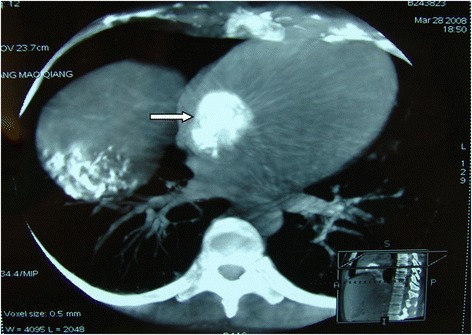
The same patient presented in Fig. 4; C-armed CT showing nice lipiodol deposition in the tumor IVC/RA thrombus after chemoembolization (→)
